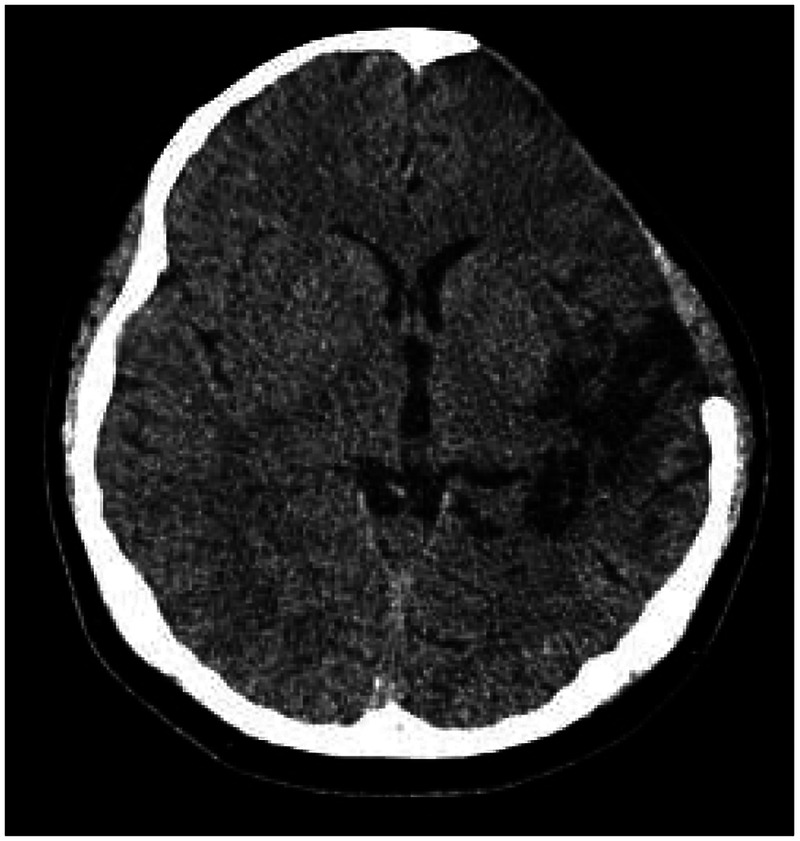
CT obtained 2 months postoperatively shows resolution of mass effect without evidence of cerebral herniation.
